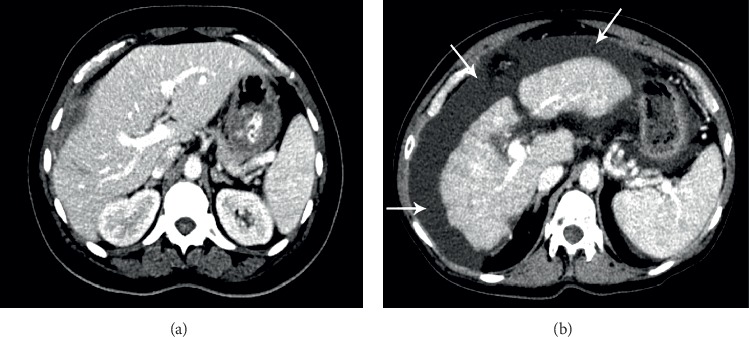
DECT and SECT of abdomen. Abdominal 120 kV second generation. (a) Effective dose adjusted for standard 40 cm acquisition length was 6.5 mSv. Images from a second-generation dual-energy CT (DECT) study in a 58-year-old male with a BMI of 26.5 kg/m^2^ demonstrating extensive ascites (arrows). (b) Adjusted effective dose was 7.0 mSv [36].
